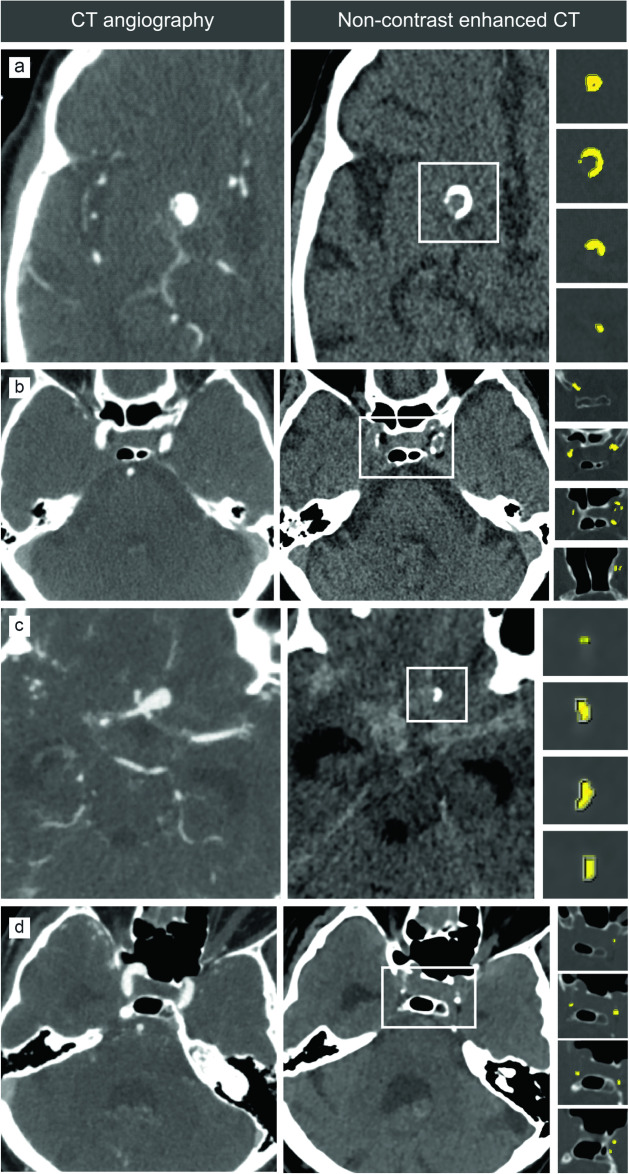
Calcification of the aneurysm wall and internal carotid arteries in representative unruptured and ruptured intracranial aneurysms. Yellow areas mark regions of interest, from cranial (top) to caudal. a CT angiography shows an unruptured 8-mm aneurysm of the right internal carotid artery tip, and non-contrast enhanced CT shows the calcified aneurysm wall (rectangle). b CT angiography and non-contrast enhanced CT show bilateral calcifications in the internal carotid arteries (rectangle). c Ruptured 10-mm aneurysm of the anterior communicating artery with a focal calcification in the aneurysm wall (rectangle). d Minimal calcifications along the course of the internal carotid arteries (rectangle)
